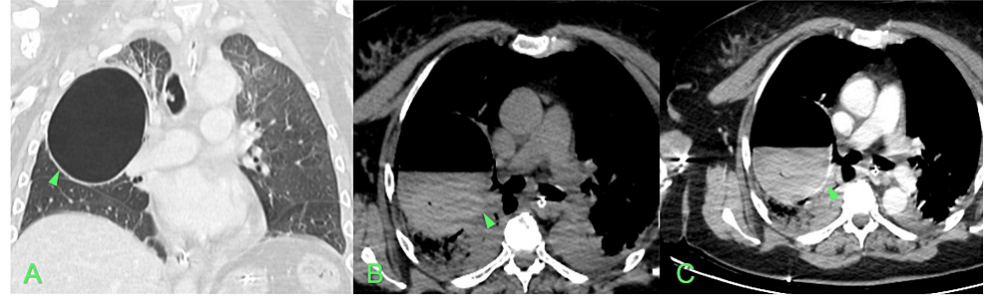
Thoracic CTA at admission with coronal cut (A) showing the emphysema bullae (arrowhead). In the axial cut, the right lung lobe is filled with heterogeneous fluid before (B) and after (C) contrast administration (arrowhead).
